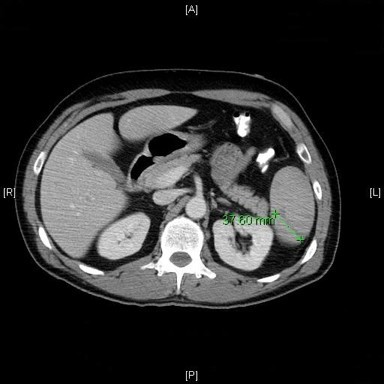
Abdominal CT scan. The CT scan from this patient shows a mildly enlarged spleen measuring 14 cm in longitudinal dimension. He had multiple splenic lacerations however, and this slice shows a 3.7 cm transverse splenic laceration.
